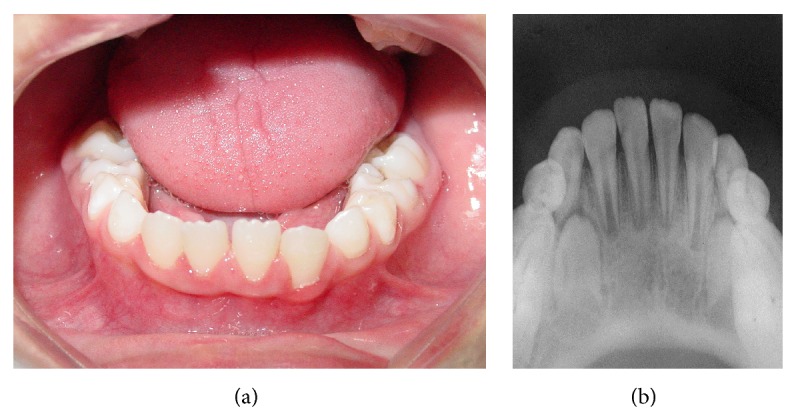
(a) Clinical aspect after removal of the splint showing the normality of soft tissues. (b) Radiographic image without splint (after 6 weeks of the trauma) indicating no pulp and periodontal pathologies.
